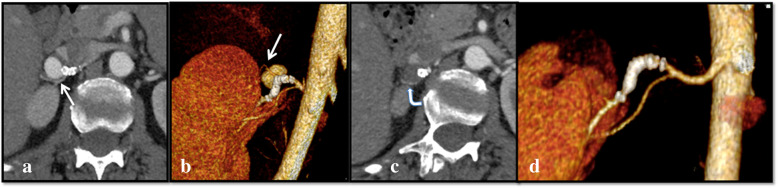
a-d Coronal CTA (a) and 3D VR imaging (b) at 6 month FU, showing a patent sidebranch (arrow), which appears smaller in comparison to baseline CT and DSA (see above Figs. 1 and 2), directed to the upper pole of the kidney. c and d show the same finding at the 12-month FU: the sidebranch ostium is occluded as well as the aneurysm. However, the distal branching vessel is still patent (curved arrow), probably thanks to collateral circulation
